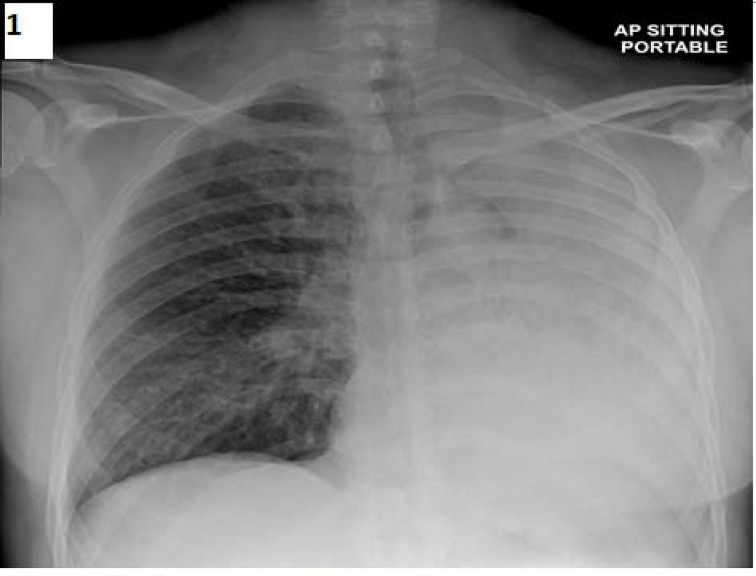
Chest x-ray showing left lung collapse
